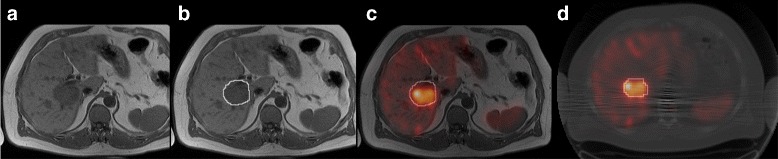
Difference for a tumour between a morphological image and SPECT. a MR image with b manually delineated morphological tumour volume. c Fusion of MR and co-registered SPECT images with morphological volume delineated. d SPECT/CT image with delineated SPECT volume using Fourier surfaces
